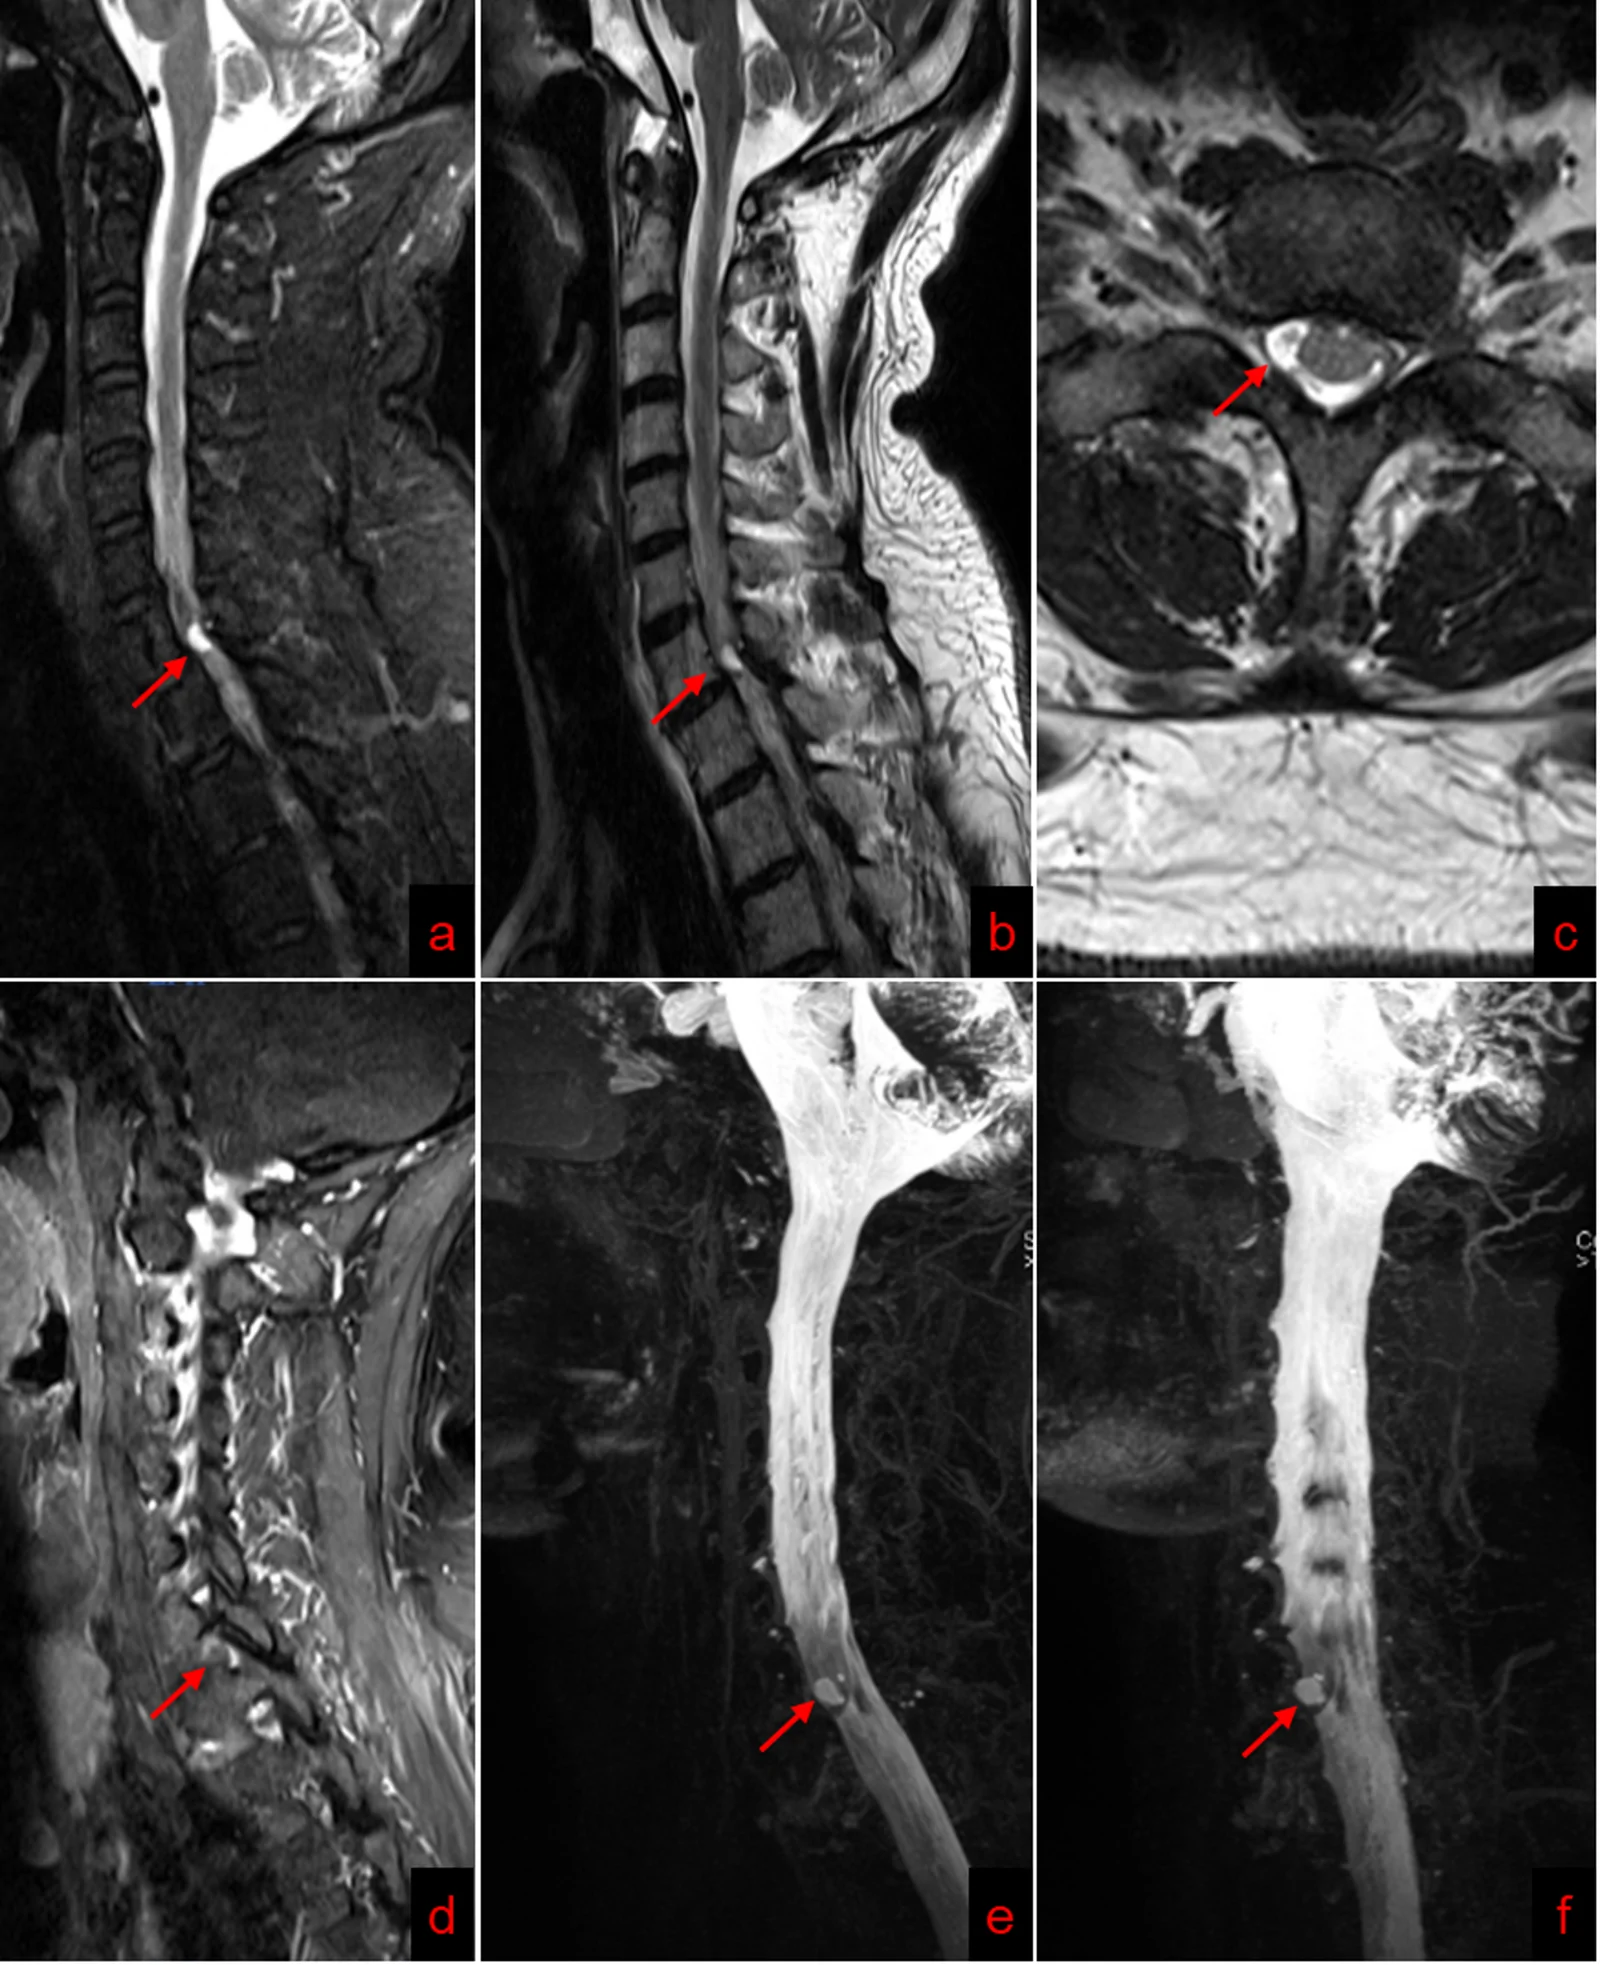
Preoperative MRI noting the C8 Tarlov cyst (red arrow). a T2-weighted fat-suppressed imaging. b, c T2-weighted imaging. d Gadolinium-enhanced MRI. e, f 3D reconstruction of T2-weighted MRI myelography
